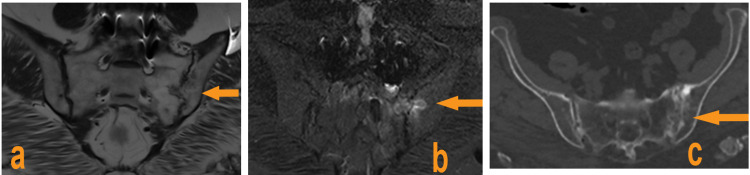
MRI sequence T1 (a), and T2 (b), and (c) CT scan of the sacroiliac joints showing sequelae sacroiliitis predominant on the left
